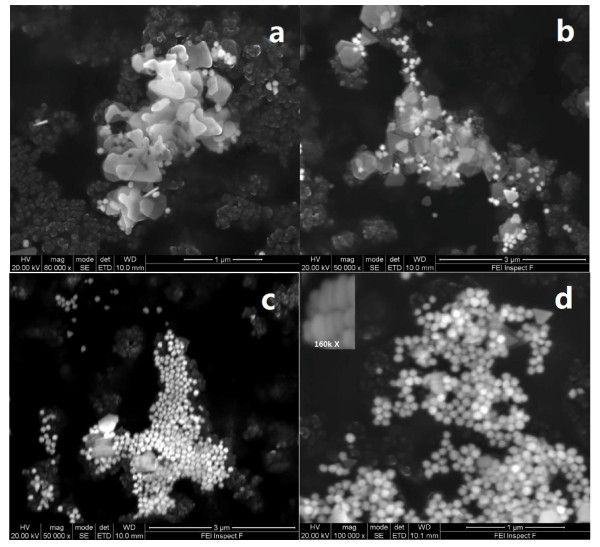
SEM images of gold nanoplates fabricated by CR with ultrasonication of 0 min. (a), 10 min (b), 20 min (c), and 30 min (d).
